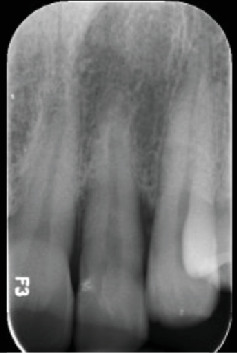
Radiographic image of the tooth revealing normal periradicular tissues with intact lamina dura surrounding the root and a uniform PDL space.
